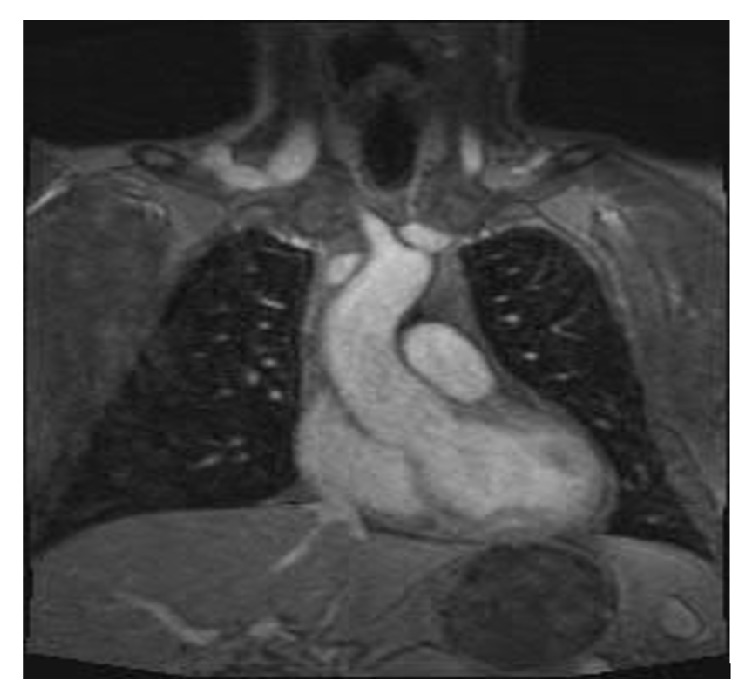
MRI of the heart with and without intravenous gadolinium contrast administration.
